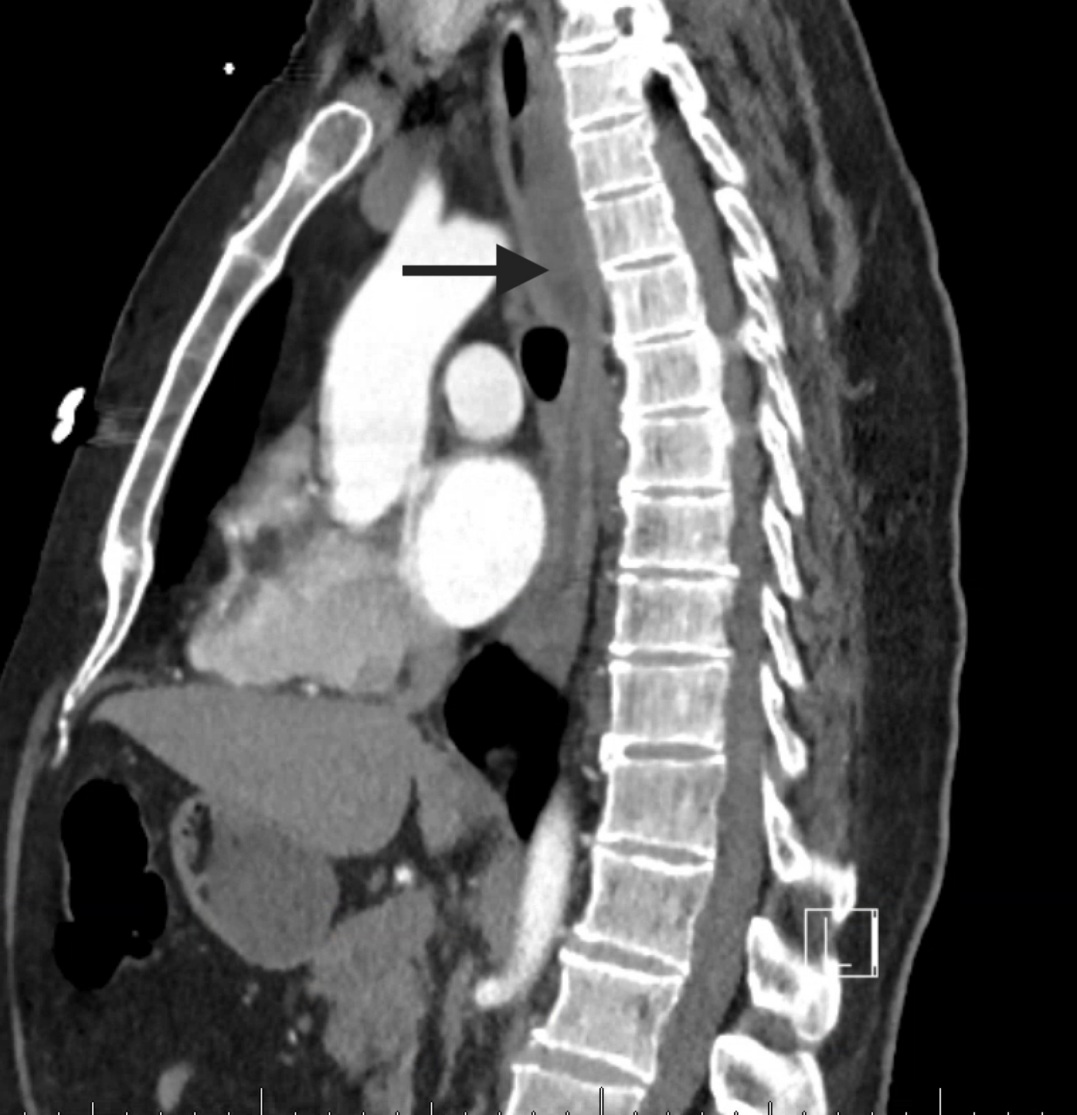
CT of the chest showing diffuse enlargement of the esophagus with small air-fluid level
